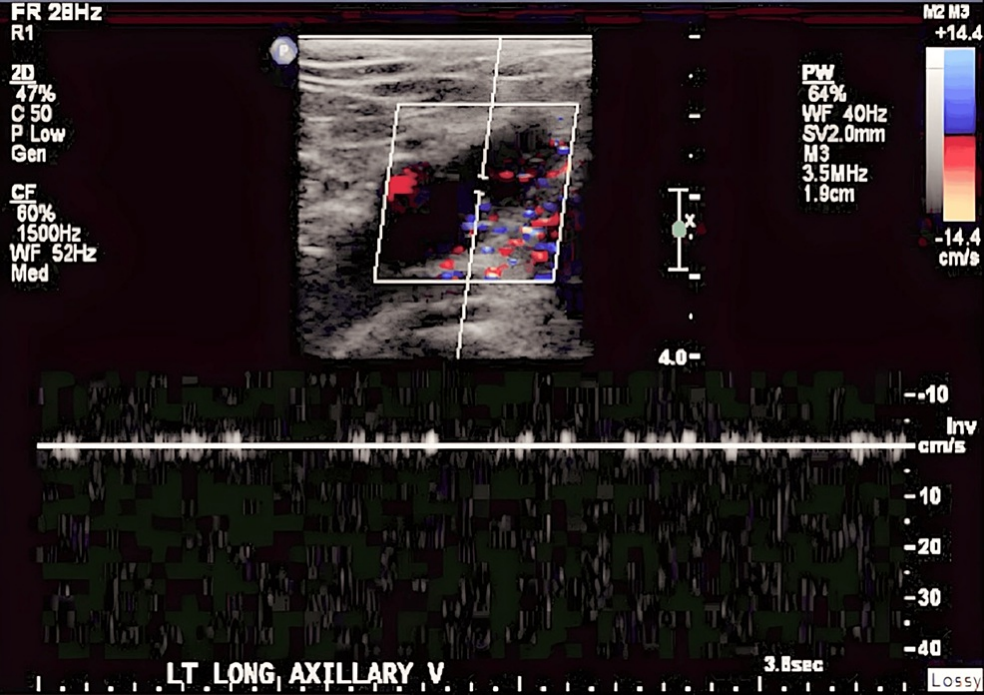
Venous Doppler of left long axillary vein showing thrombosis
